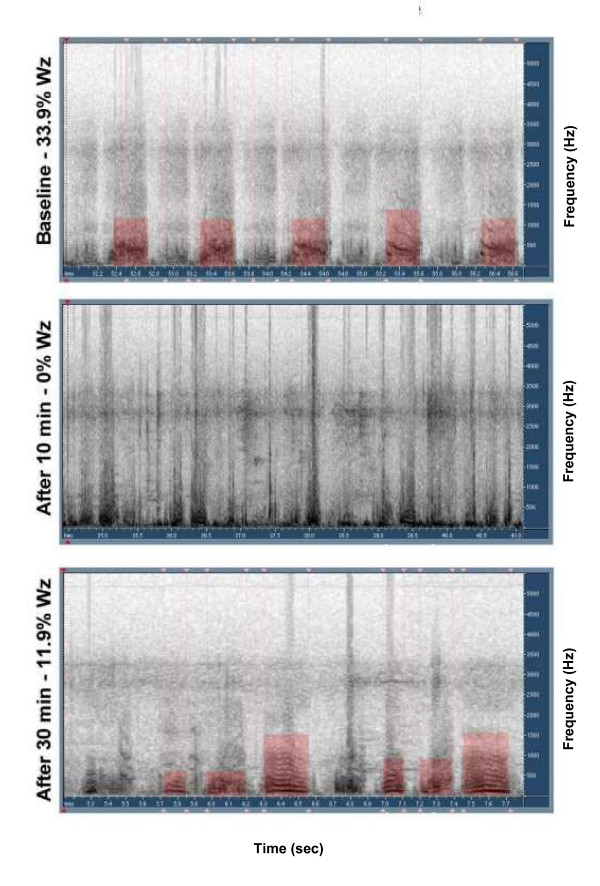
Sonogram of subject treated with epinephrine showing transient response with relapse.
